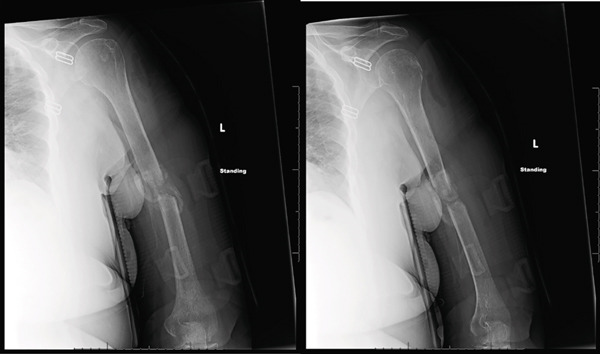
AP and lateral radiographs of the left humerus taken 12 weeks after injury demonstrating a nonunion.
